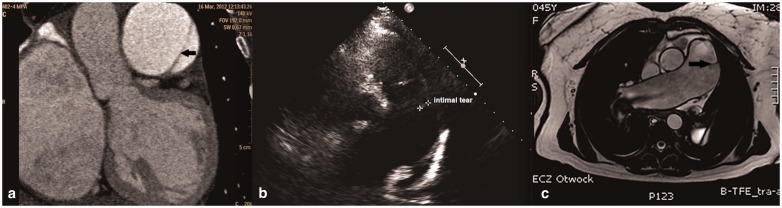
(a) CT, (b) echocardiography, and (c) MRI all revealed the intimal tear (black arrows) which began 1.5–3 cm beneath the pulmonary valve and was 3.5 cm long. The dissection extended slightly beyond the bifurcation of the main PA.
